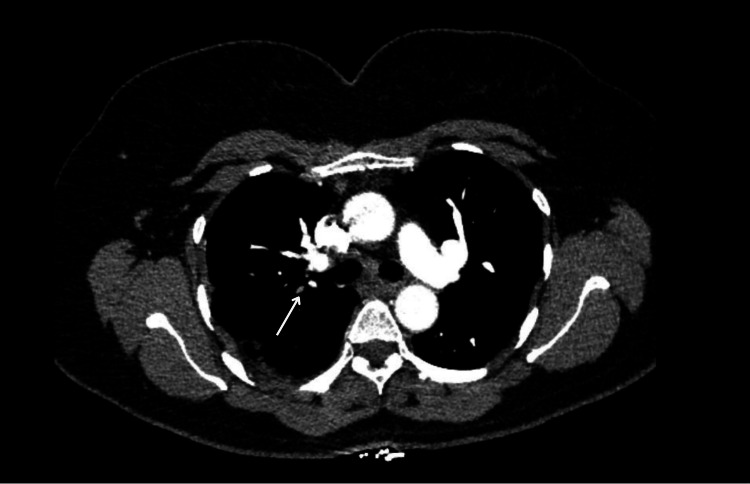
Axial CT pulmonary angiography (CTPA) image from Case 2 demonstrating a small filling defect within the segmental venous branches of the right upper lung lobe (arrow)
